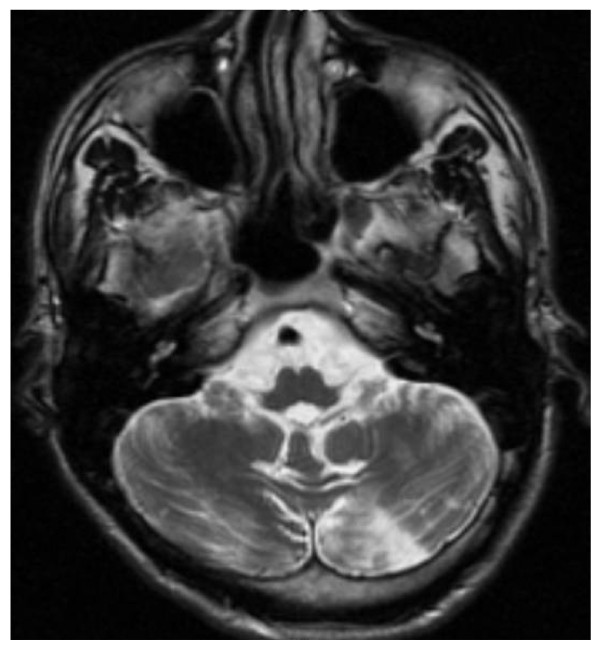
Stroke in a patient affected with Fabry disease: axial brain MRI section showing stroke of the left cerebellar hemisphere that revealed Fabry disease in an otherwise asymptomatic 27-year-old male patient.
